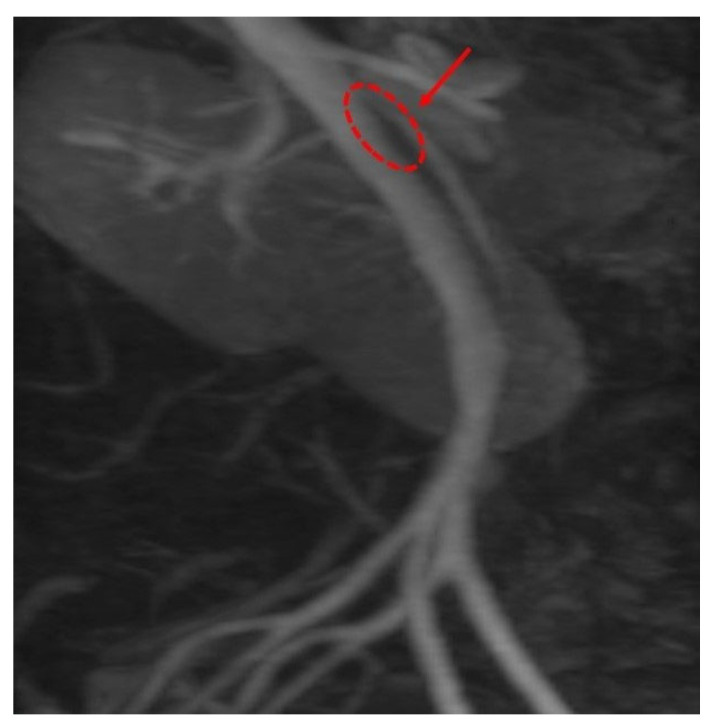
CTA: Findings consistent with superior mesenteric artery SMAS.
